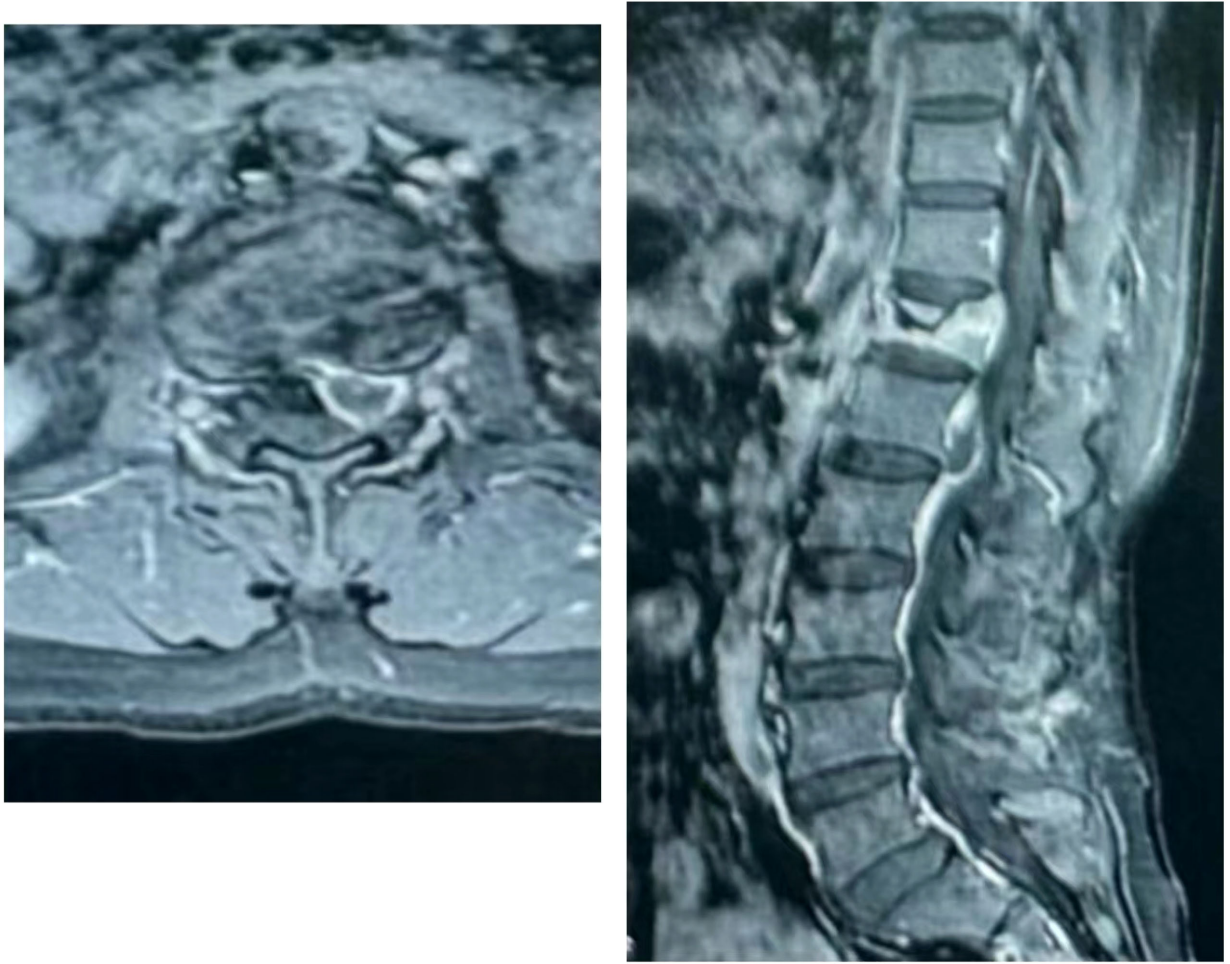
The 5-year postoperative follow-up CT and MRI showed good healing of the T12 vertebral body fracture, with no evidence of bone destruction in the remaining vertebral bodies or their appendages. Additionally, no signs of recurrent soft tissue ma.
